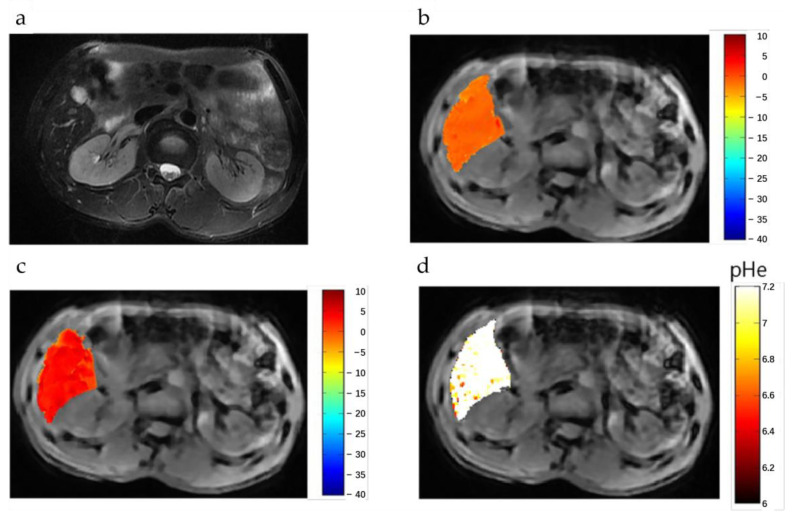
pHe values of hepatic hemangioma using dual-power CEST MRI. (a) A representative image of a patient with hepatic hemangioma.After injection of a CT agent (Ioverol), CEST images acquired with flip angles of 60° (b) and of 350° (c), respectively. (d) The pHe map for hepatic hemangioma; the CEST pHe was consistent with the surrounding liver tissue, confirming the hemangioma to be benign. Reproduced with permission from Frontiers 2020 (DOI: 10.3389/fonc.2020.578985) [72].
